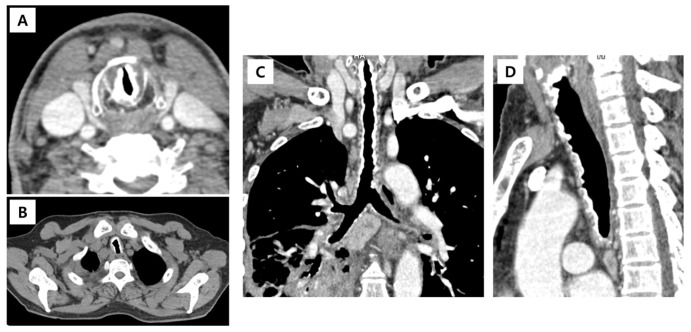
Computed tomography (CT) images of the airway in a patient with tracheobronchopathia osteochondroplastica. (A) Axial CT image showing marked calcification at the level of the vocal cords; (B) axial CT image demonstrating calcification of the upper trachea; (C) coronal CT reconstruction revealing diffuse osteocartilaginous calcification throughout the trachea and main bronchi; (D) sagittal CT reconstruction showing diffuse osteocartilaginous calcification involving the trachea and bronchi.
